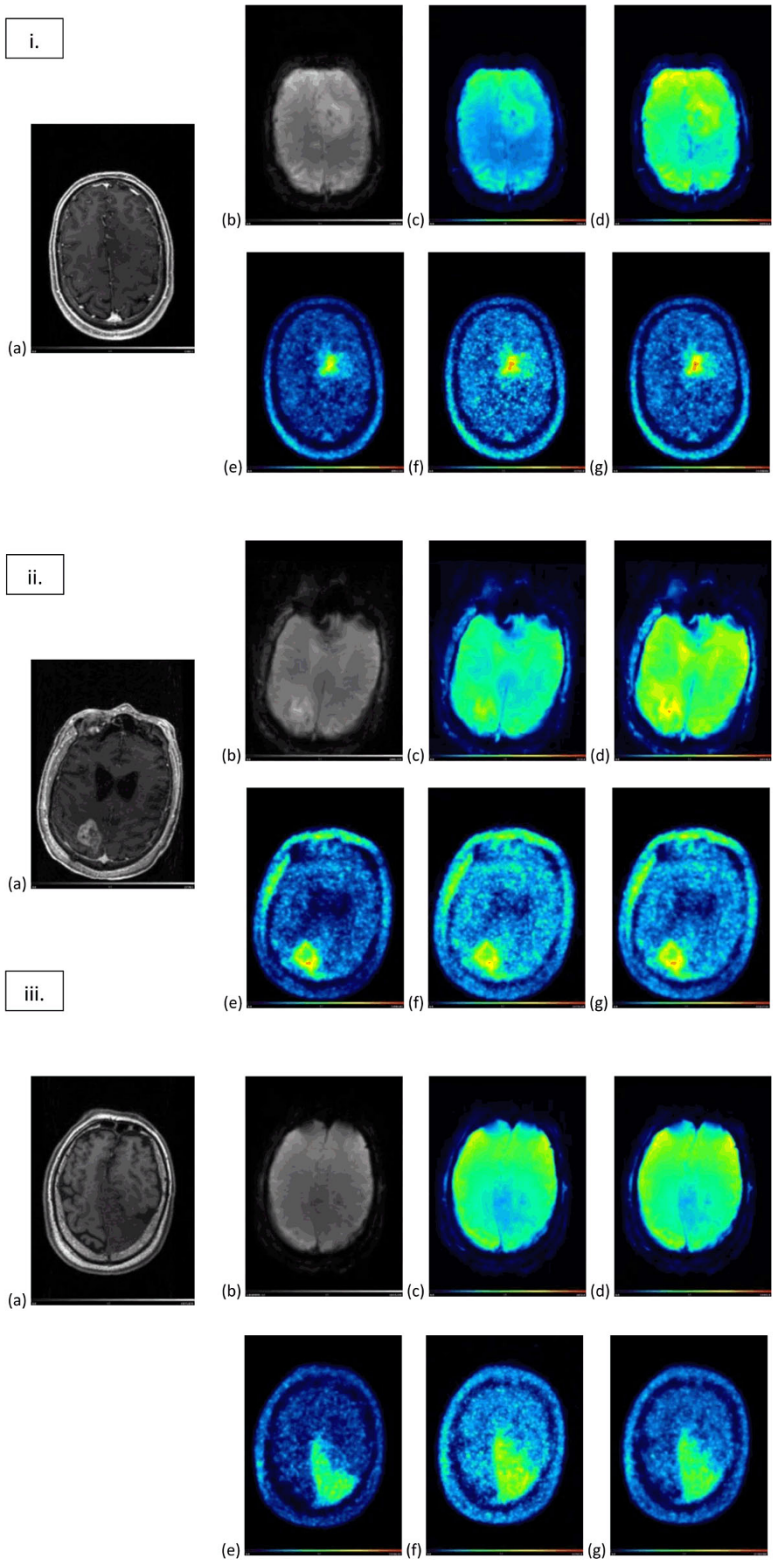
Analyzed datasets (i, ii and iii). MRI images (a) MPRAGE (post contrast), (b) DSC-MRI EPI, and the corresponding extracted parametric images: (c) peak and (d) AUC. (e) FET-PET image (summed image of 20-40 min p.i., previously filtered with a 2 mm Gaussian filter) and the corresponding extracted parametric images of (f) peak and (g) AUC.
